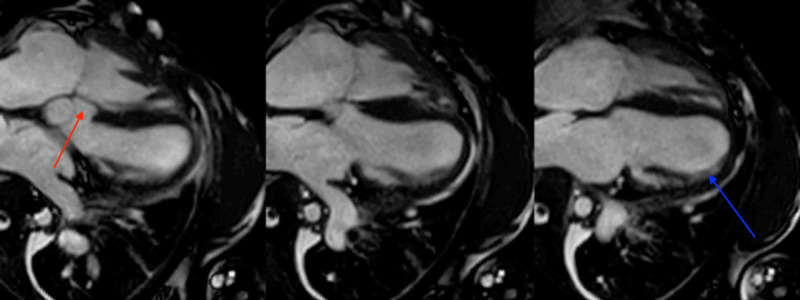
Ventricular Septal Defect MRI imaging displays a defect in the interventricular septum (red arrow). Imaging also shows evidence of right ventricle (RV) enlargement (blue arrow).
